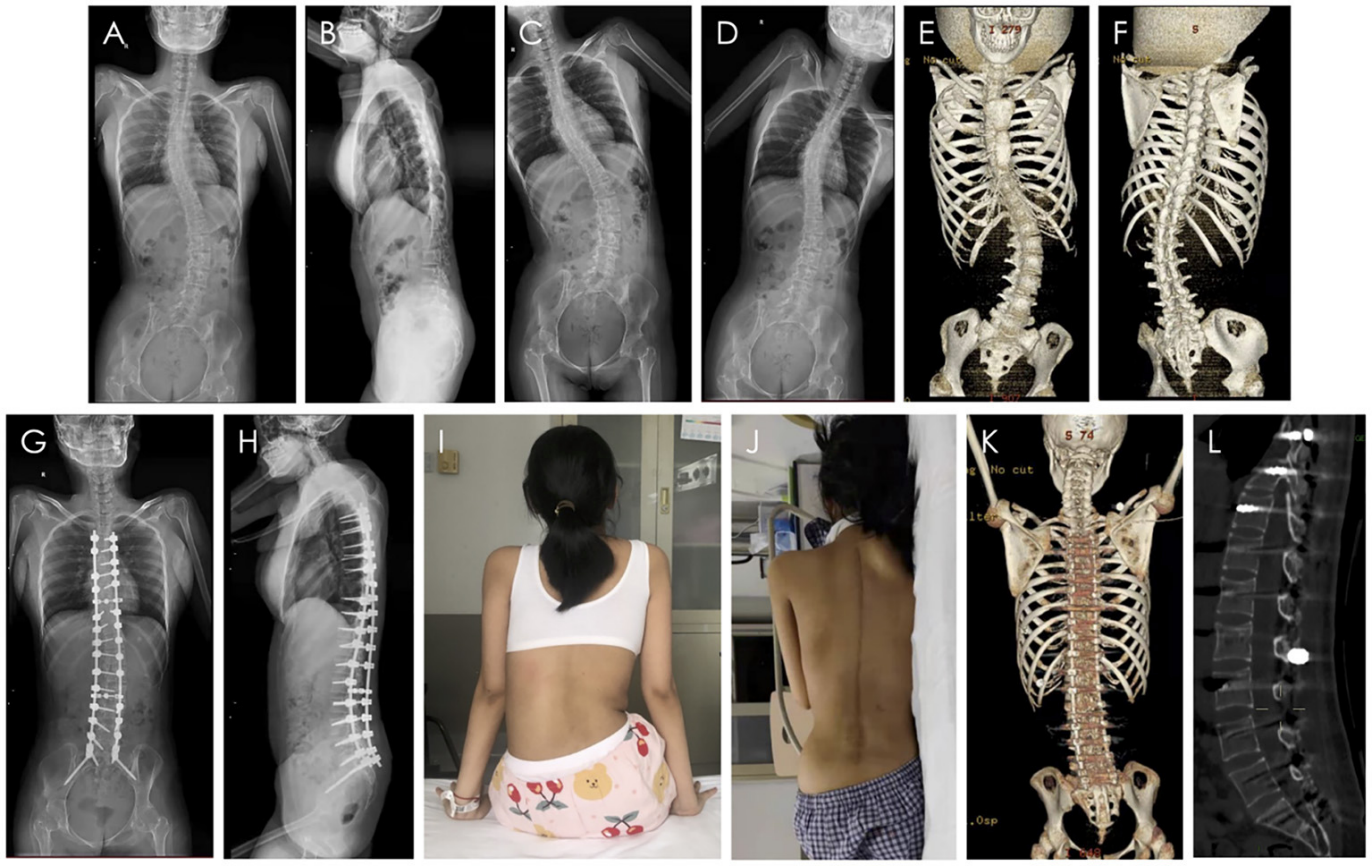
Scoliosis orthopedic surgery. X-ray, CT images and appearance of the SMA patient with scoliosis. (A) The anteroposterior view of spinal X-ray before surgery. (B) The lateral view of spinal X-ray before surgery. (C,D) The left and right bending view of spinal X-ray before surgery. (E,F) The three-dimensional CT images before surgery. (G) The anteroposterior view of spinal X-ray after surgery. (H) The lateral view of spinal X-ray after surgery. (I) The appearance of the back before surgery. (J) The appearance of the back after surgery. (K) The three-dimensional CT images after surgery. (L) The sagittal CT images after surgery and the osseous channel are circled in the figure.
